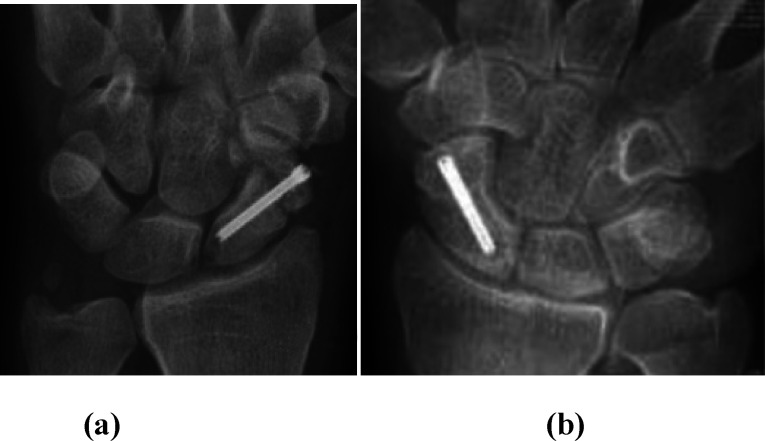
a) Preoperative PA wrist X-ray of no-nunited scaphoid fixed with Herbert screw. b) Follow-up X-ray showing full union after revision fixation using MFC graft.
